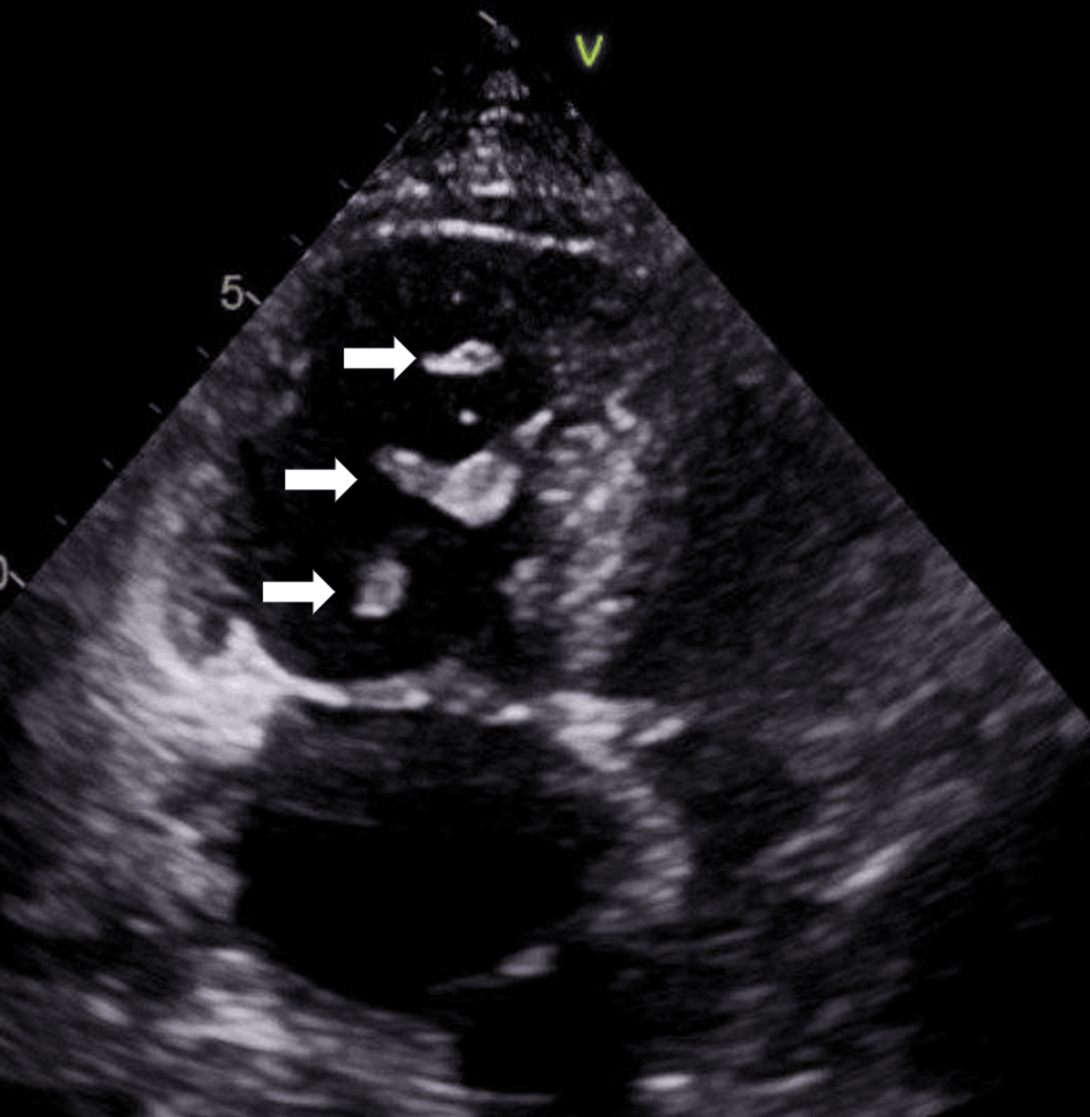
TTE shows an apical four-chamber view (RV focus) showing a thrombus in the RV (white arrows). RV: right ventricular; TTE: transthoracic echocardiogram
